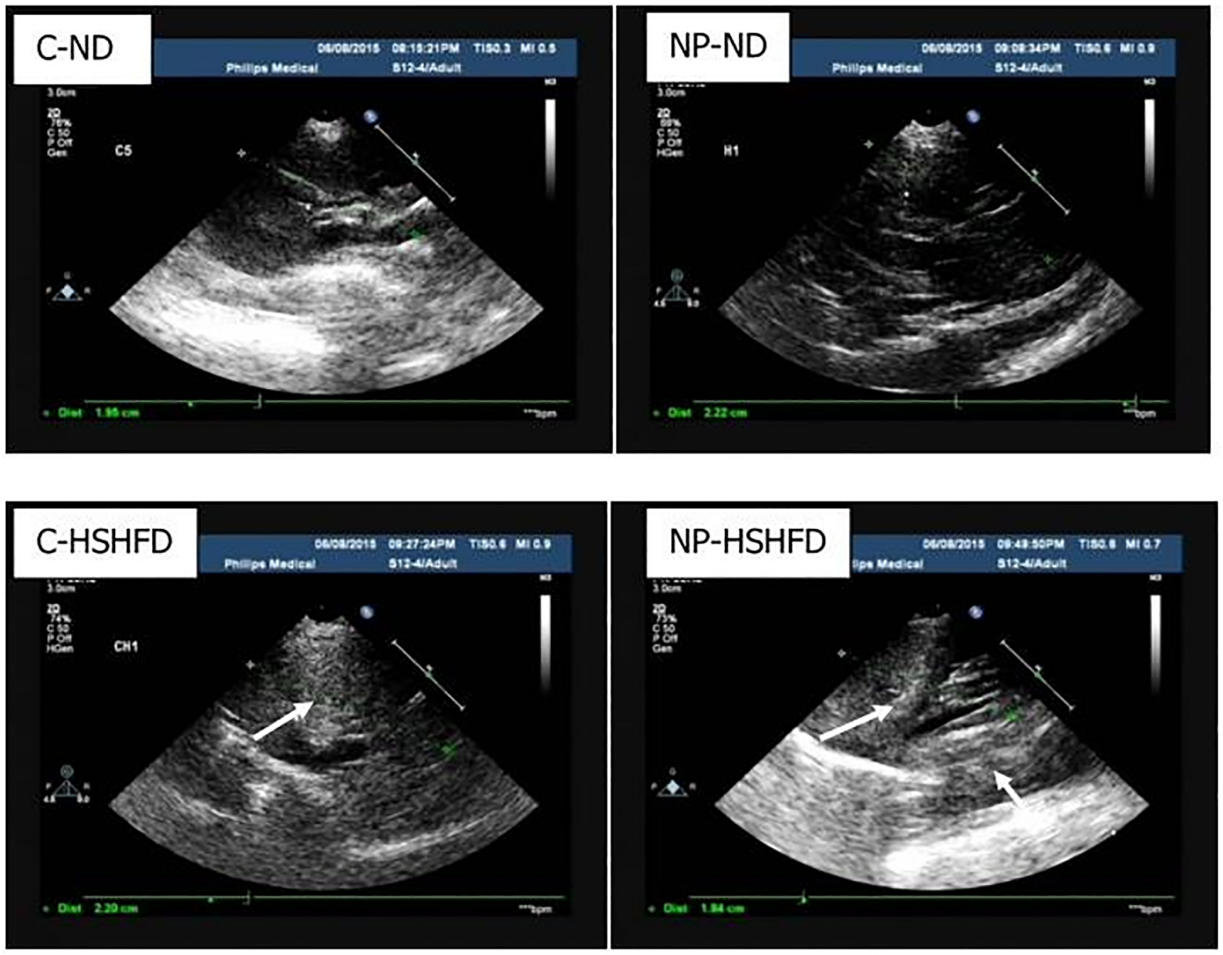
Liver ultrasound imaging. The hyperechogenicity of liver parenchyma (arrowheads). C-ND: normal-diet control; NP-ND: normal-diet plus NP; HSHFD: high-fat-diet control; NP- HSHFD: high fat diet plus NP.
